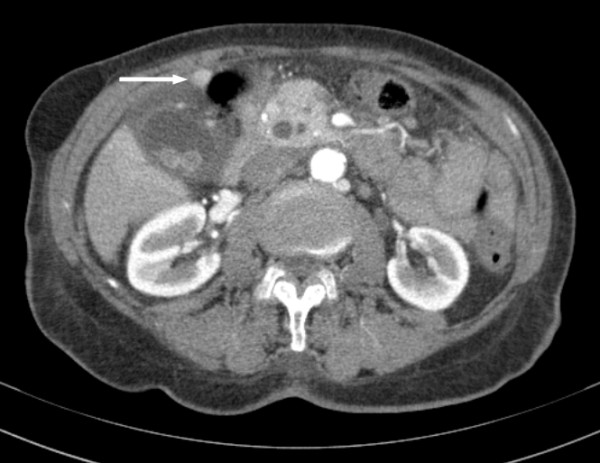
Transversal CT scan, showing the ectopic liver (arrow).
